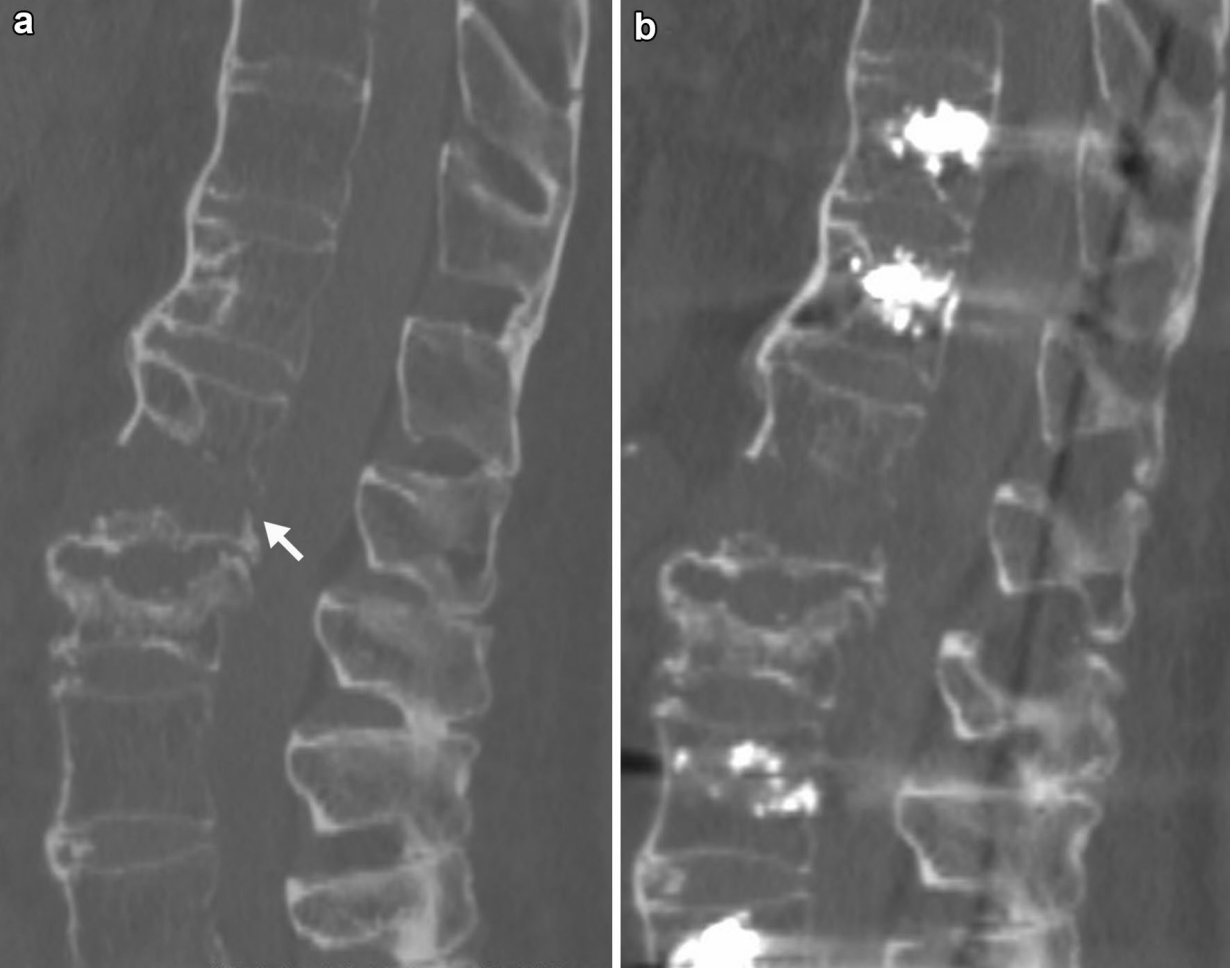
79-year-old male, hyperextension injury of Th12 (above a pre-existing, healed compression fracture of L1), 21° of lordotic angulation in the pre-operative CT scan (a). Postoperative CT scan prior to mobilisation after cement-augmented percutaneous less rigid posterior instrumentation. No relevant intraoperative reduction of the lordotic angulation (b)
